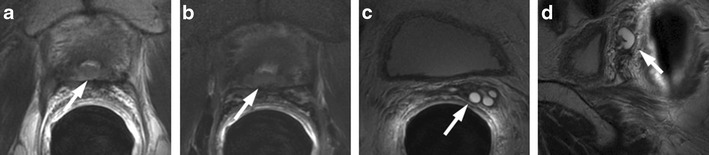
MR images of a 63-year-old man with prostate-specific antigen progression (PSA serum level 1.9 ng/mL) after radical retropubic prostatectomy. a Axial T2-weighted fast spin-echo (6006/109) and b axial T2-weighted fat saturated fast spin-echo image showing residual glandular tissue. c Axial T2-weighted fast spin echo (6006/109) and d sagittal T2-weighted fast spin echo (5059/120) showing retained seminal vesicles, which appear as high signal intensity tubular structures (*white arrows*)
